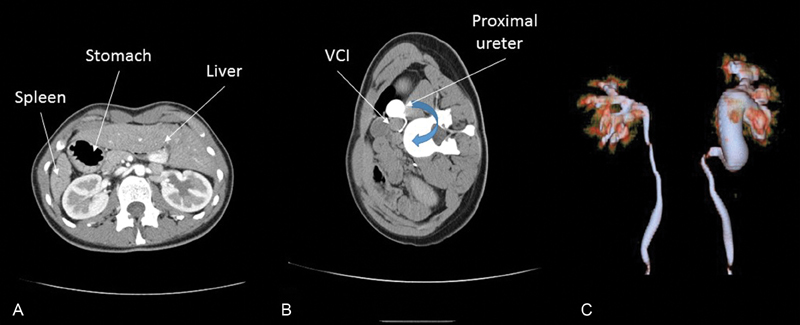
Abdominal computed tomography scan with contrast injection. (
